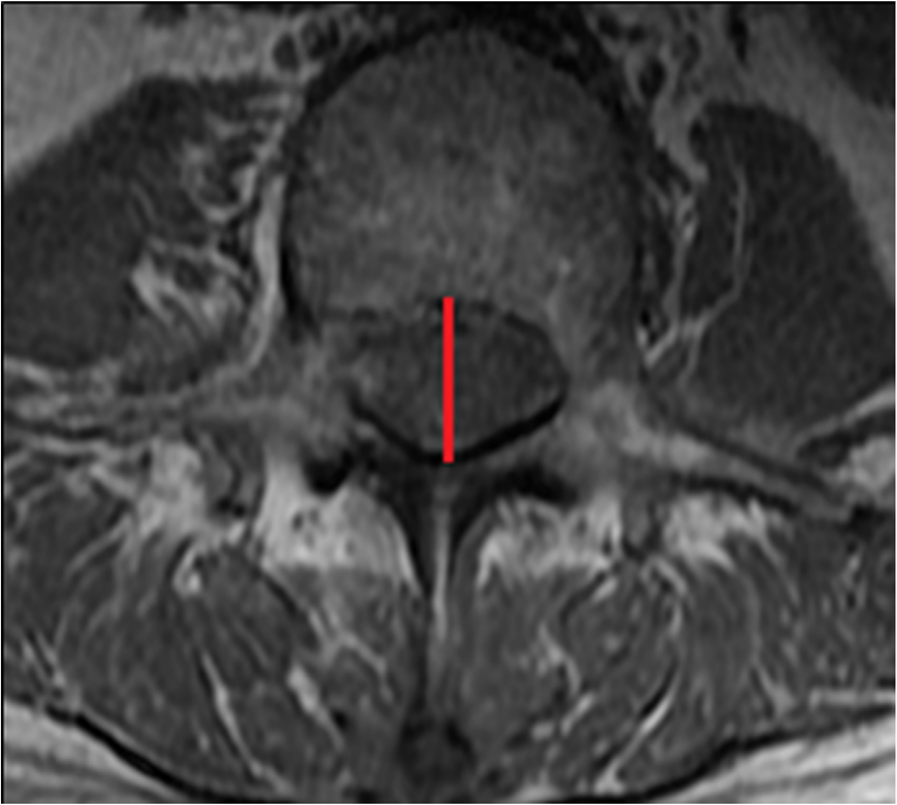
Axial T1 MRI image showing the measurement for the anteroposterior bony spinal canal diameter
